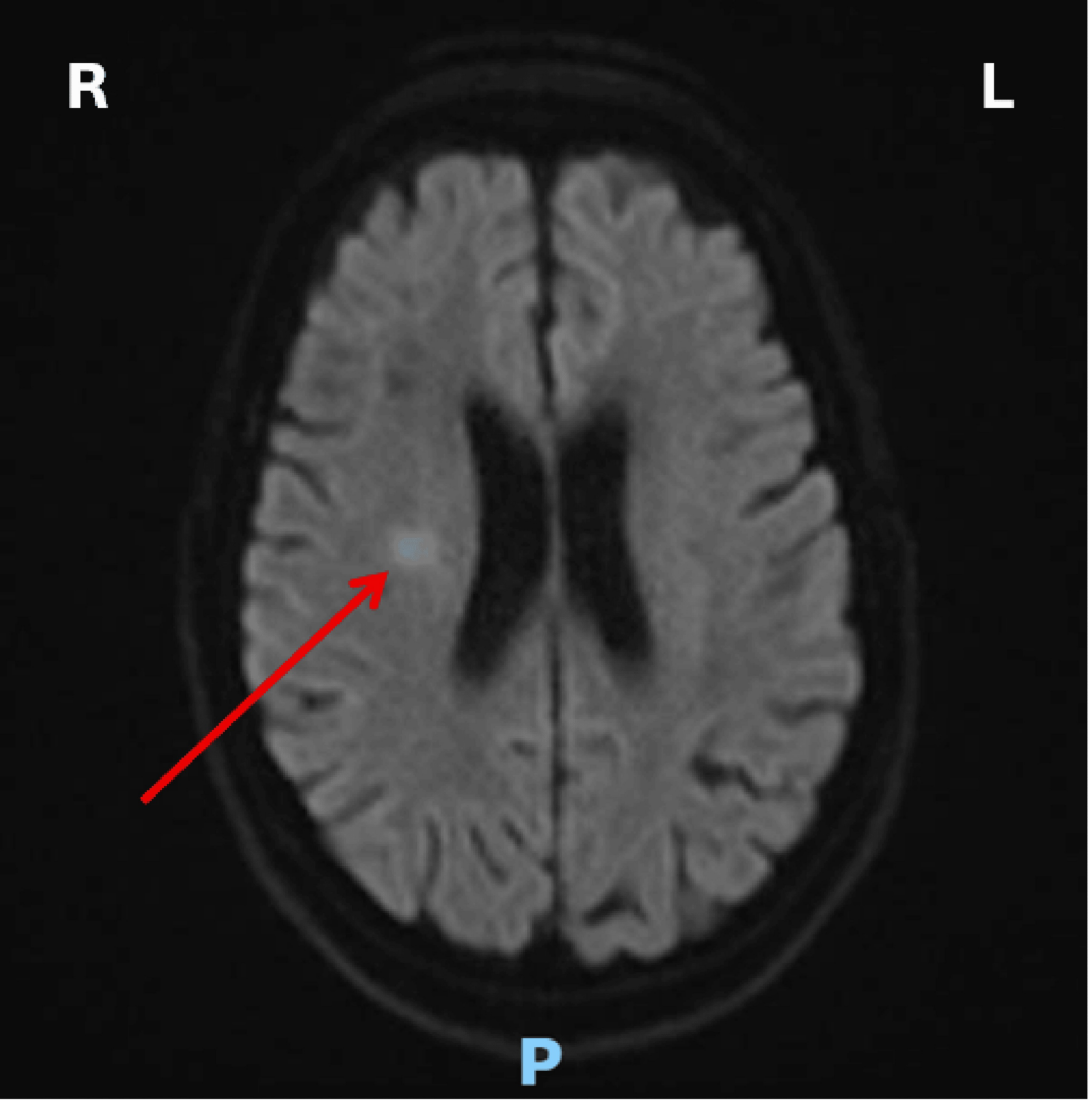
Axial MRI brain DWI demonstrating an acute infarct (red arrow) MRI: magnetic resonance imaging; DWI: diffusion-weighted imaging MRI brain showing a small acute infarct in the white matter of the right frontal lobe
